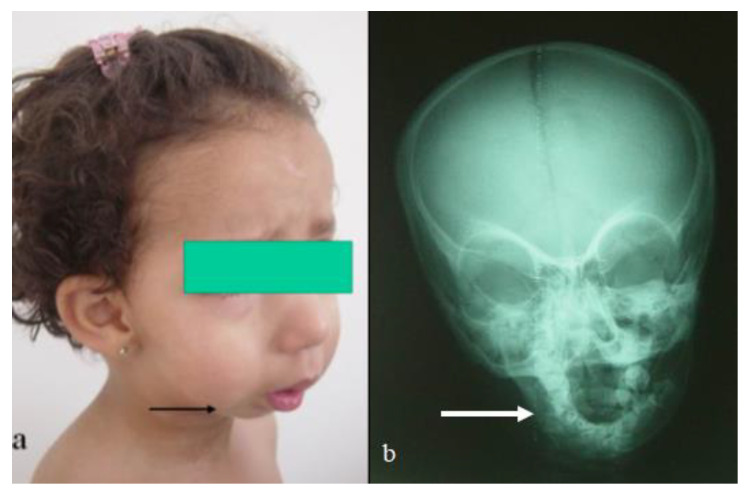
Clinical photo of a 4-year-old- girl with progressive hemi-atrophy of the right side of the face, which shows apparent unilateral facial atrophy of the right side of the face (arrow) (a); AP skull radiograph showed apparent atrophy of the right side of the mandible, giving rise to unilateral micrognathia (right sided atrophy of the maxilla, and the alveolar process of the maxilla) (arrow) (b).
